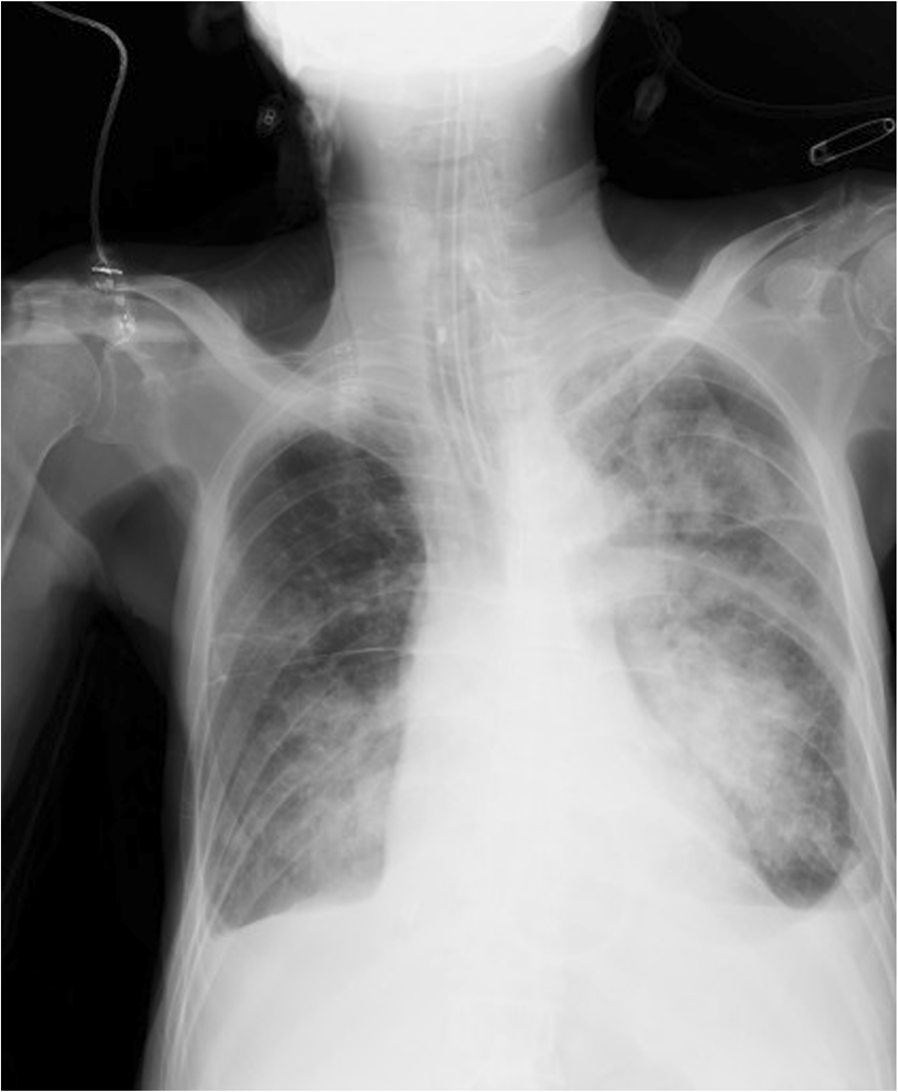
Chest X-ray depicting diffuse bilateral pulmonary infiltrates after tracheal intubation on intensive care unit day 1
